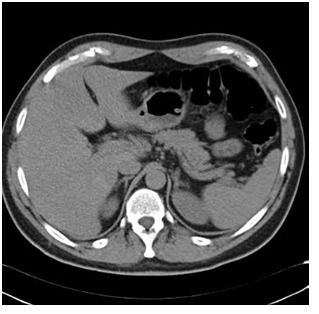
Computed tomography imaging of the adrenal glands
